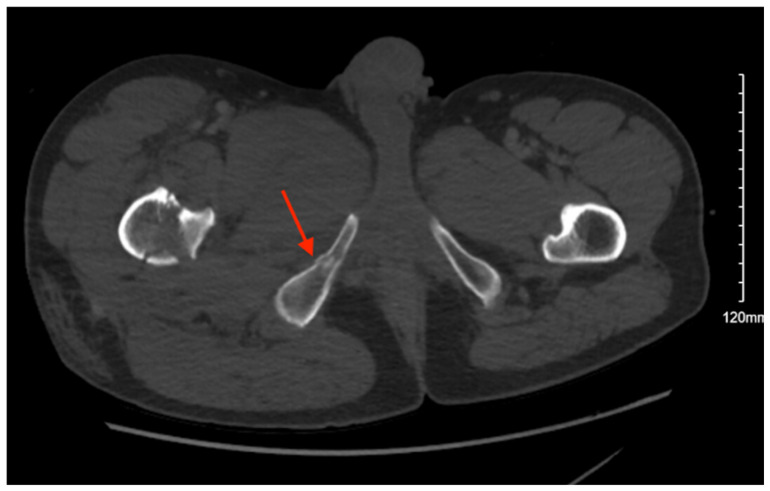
CT abdomen/pelvis images of the described fracture in case 7. Red arrows are used to indicate the location of pelvic fractures for easier identification.
